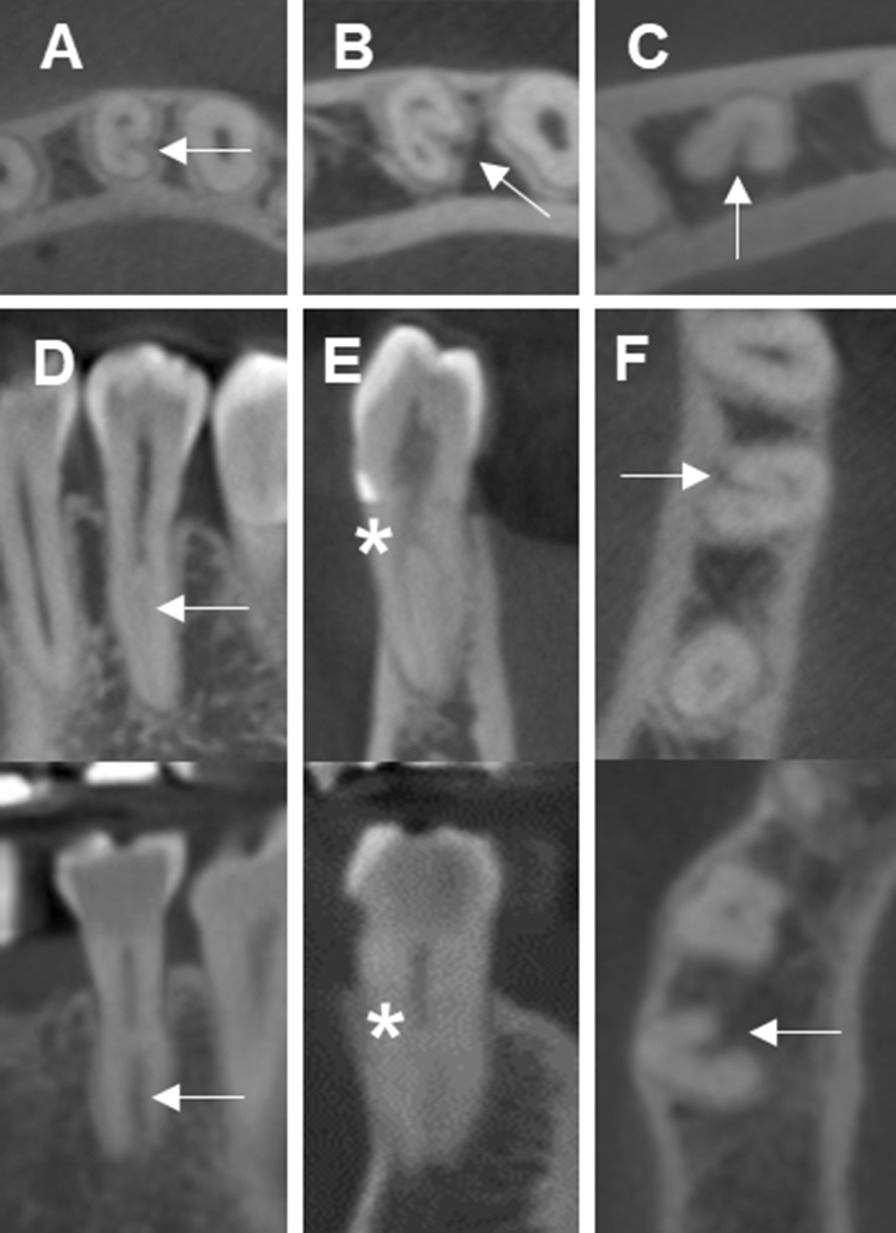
A–C Axial view of CBCT images show the location of radicular grooves in C-shaped root canals in A Mesial, B Mesiolingual, and C Lingual aspects of the root. D Sagittal view of CBCT images show a radiolucent longitudinal line between the mesial and distal part of the root. E Coronal view of CBCT images show single-rooted premolars with 1 root canal extending from the pulp chamber and dividing into 2 root canals. F Axial view of CBCT images show C-shaped root canals with a lingual radicular groove. (Arrows indicate radicular grooves; asterisks indicate where the root canal divides from 1 into 2 root canals)
